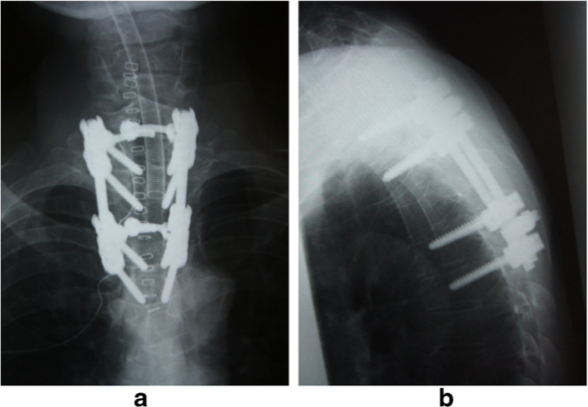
Postoperative radiographs. Postoperative anterior-posterior (a) and lateral (b) radiographs showing T1-T5 pedicle screw instrumentation.
